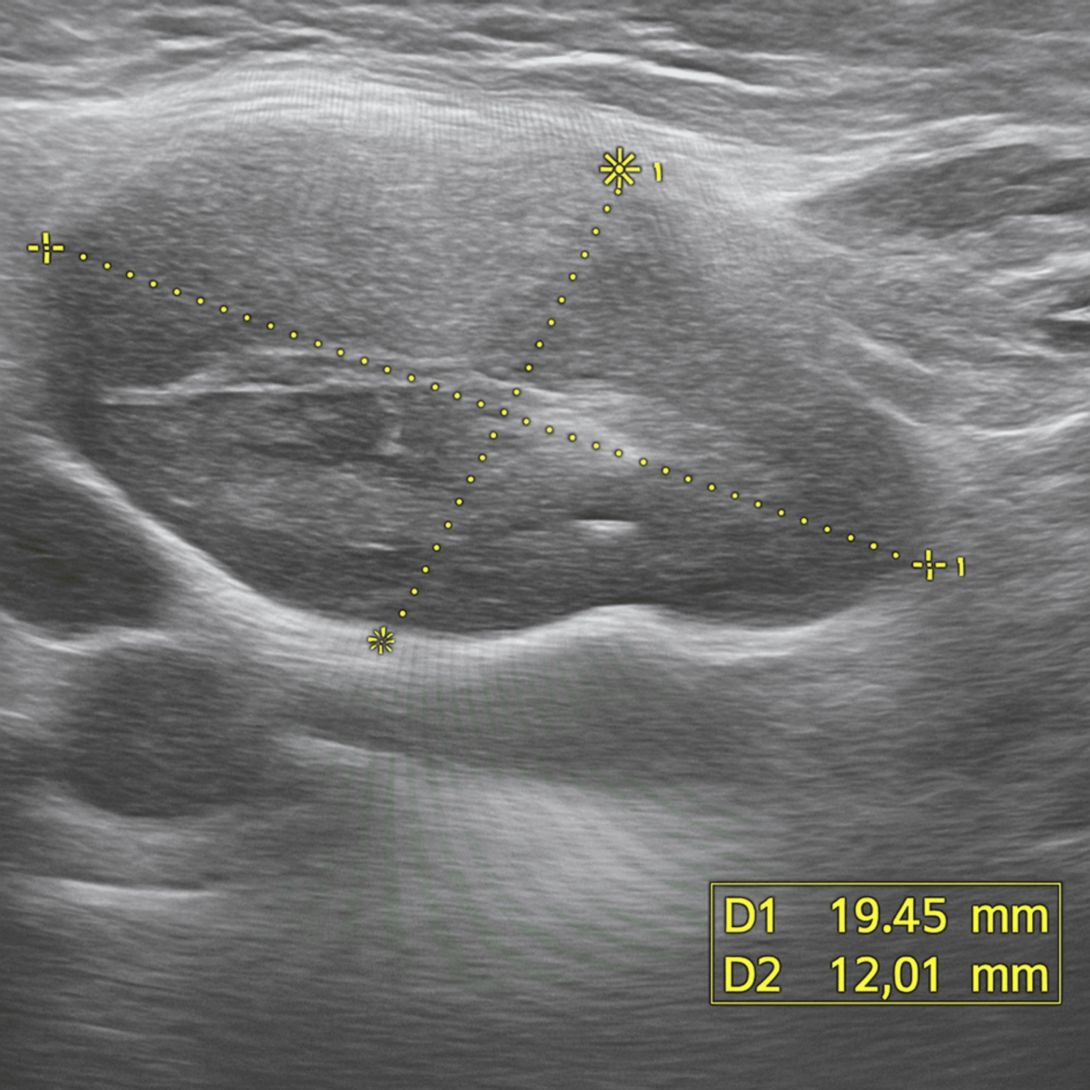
Ultrasound image of the largest cervical lymph node, showing features suggestive of benignity
